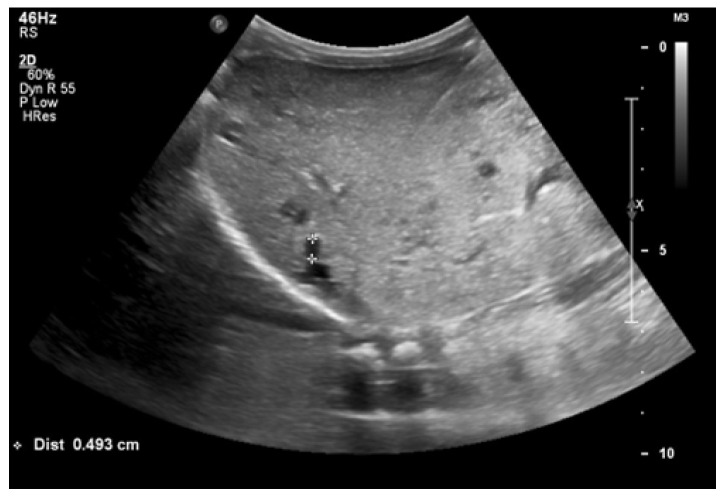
Ultrasonography of the liver at the age of four months (hyperechogenic, enlarged liver with multiple cysts).
